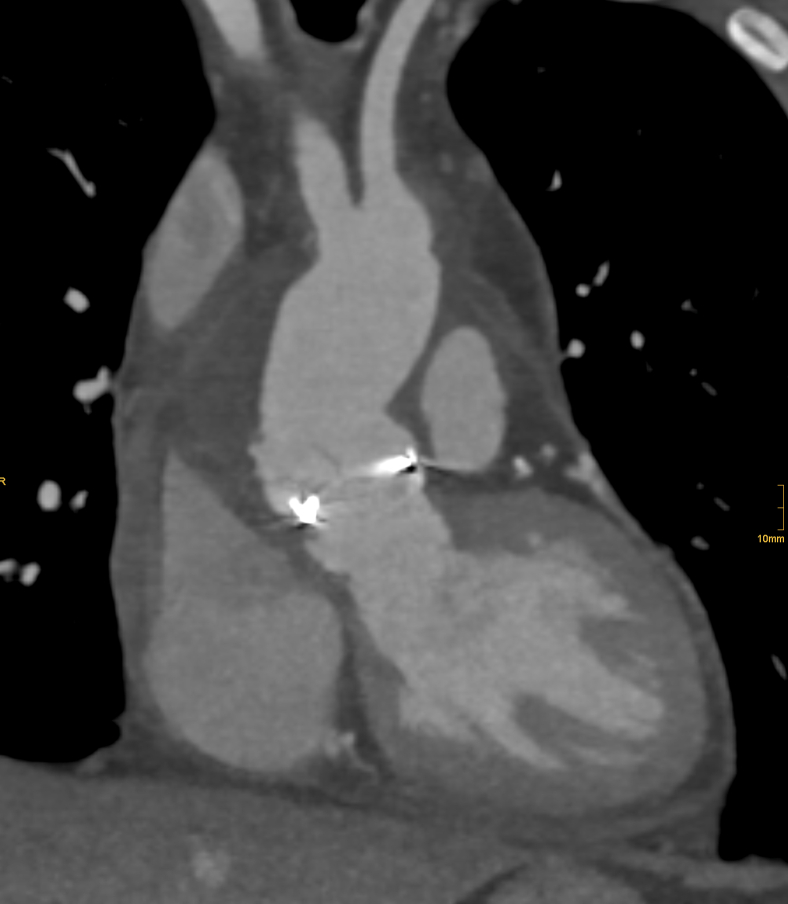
Frontal plane of postoperative CT scan after aortic valve replacement and A. Kocher root enlargement.
